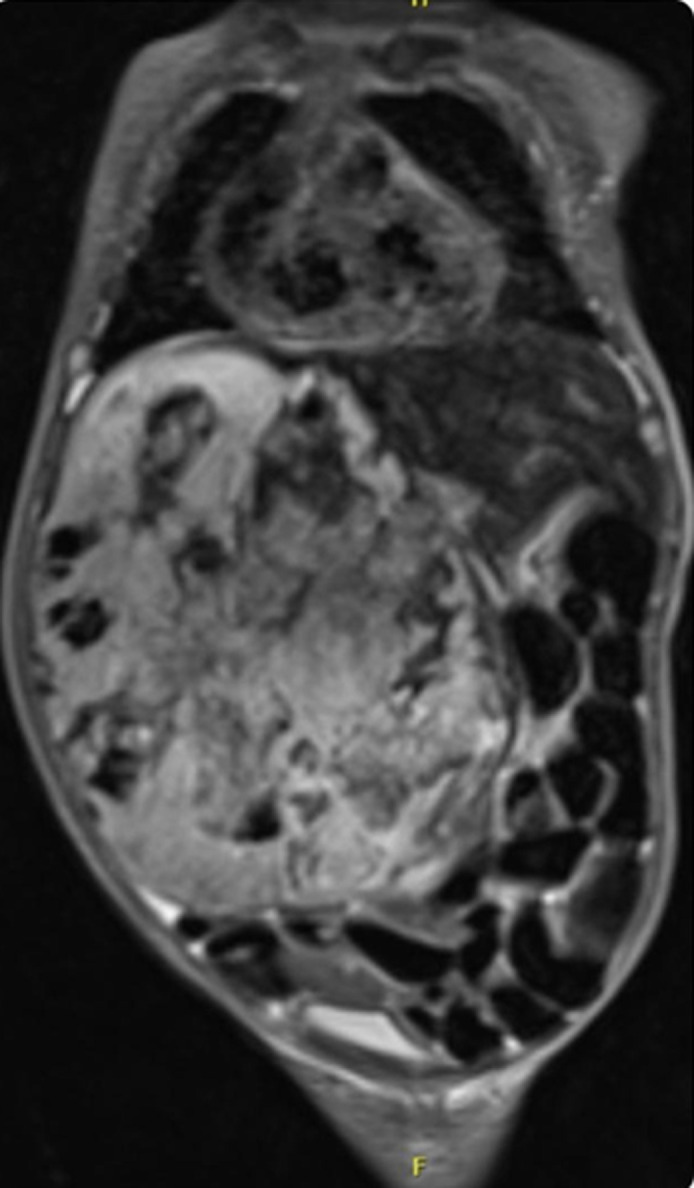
MRI abdomen showing large exophytic T2 heterogeneous mass with hypointense areas arising from the anterior segments of right and left lobe of liver, occupying entire right side of abdomen
